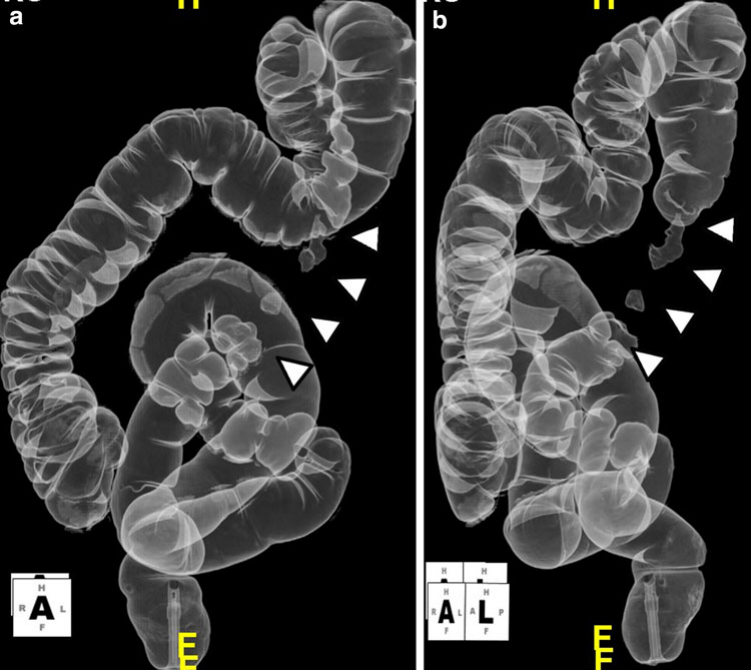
Dorsal position (a) and left anterior oblique (b) of CT colonography. The descending colon became intermittently blocked by the hematoma (*arrow*)
